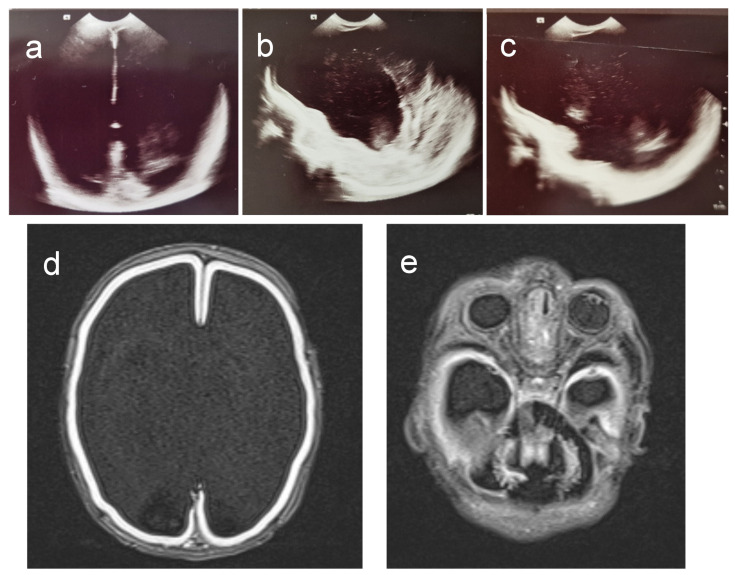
Brain ultrasound and MRI. (a–c). Brain ultrasound demonstrates dilated cerebral hemispheres, thin mantle of brain tissue on the convexity, absent basal ganglia and hypoplastic pons, and cerebellum. (d,e) Brain MRI showing complex anomalies consistent with WWS. (a) Coronary plane. (b) Medio-sagittal plane. (c) Parasagittal plane. (d) Supratentorial. (e) Infratentorial.
